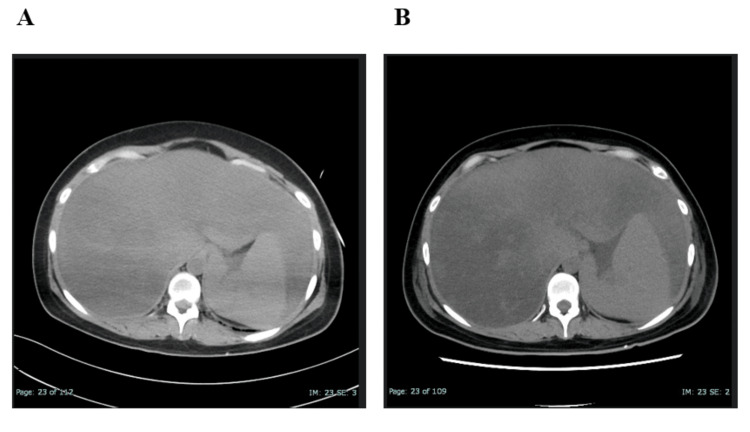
Non-contrast CT image of the upper abdomen Figure 1A was obtained at the time of cesarean section, and Figure 1B at the time of transfer to our ICU. In both images, the liver-to-spleen attenuation ratio was below 0.9, indicating fatty liver. The hepatic attenuation in Figure 1B was further reduced compared to Figure 1A. CT: Computed tomography
